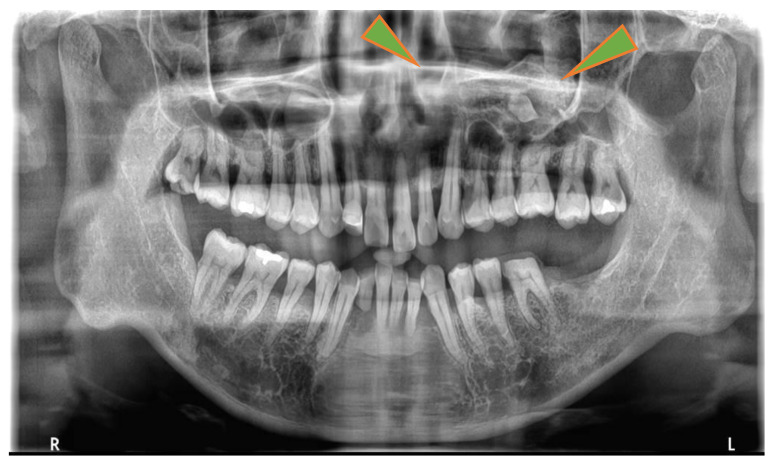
Orthopanoramic radiographs. A well-circumscribed radiopaque is observed in the upper jaw sinus. No permanent tooth deficiency in the maxillary dentition was noted, and wisdom teeth had erupted.
